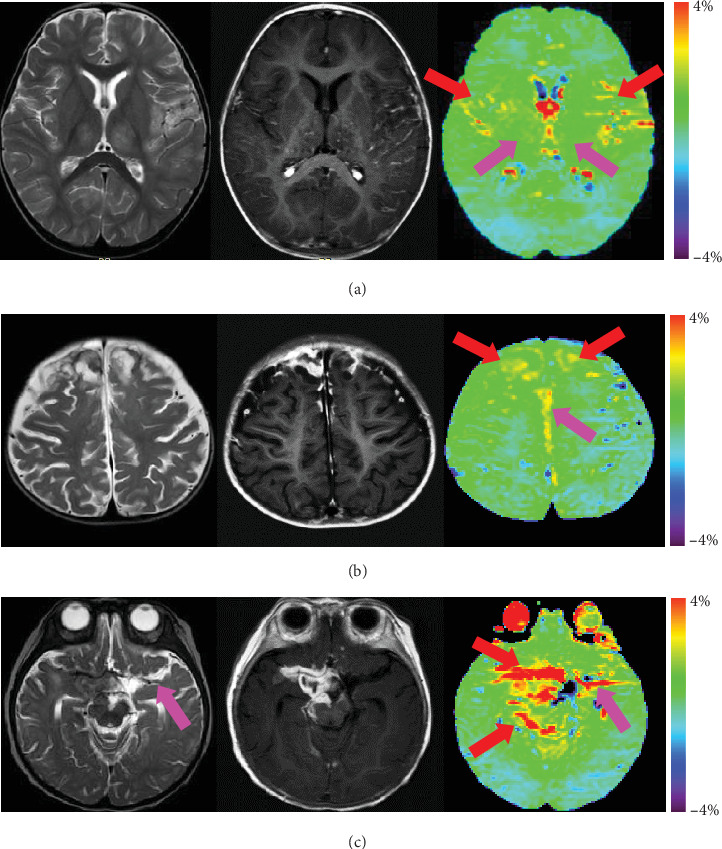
APTw and conventional MR images for three patients with meningitis. (a) T2w, Gd-T1w, and APTw images for a 17-month-old girl with viral meningoencephalitis. T2w image demonstrates symmetric increased signal intensity involving bilateral thalami and temporal lobes. The Gd-T1w image shows slight spotted enhancement in bilateral thalami and leptomeningeal enhancement in the bilateral temporal regions. The gadolinium-enhancing lesions (pink arrows) and leptomeninges (red arrows) show slight hyperintensity on the APTw image. (b) T2w, Gd-T1w, and APTw images for a 9-month-old girl with pyogenic meningitis. Gd-T1w image demonstrates leptomeningeal enhancement in the frontal regions and adjacent cerebral falx. Consistent with Gd-T1w, the APTw image shows hyperintensity in the frontal regions (red arrows) and adjacent cerebral falx (pink arrow). (c) T2w, Gd-T1w, and APTw images for a 7-month-old boy with tuberculous meningitis. Gd-T1w image shows enhancing subarachnoid space lesions in the right basilar cisterns, with extension into right sylvian vallecula, ambient cistern, and quadrigeminal cistern. The enhancing leptomeninges also show hyperintensity on the APTw image (red arrows). Note the APTw hyperintensity in the left sylvian vallecula and temporal lobe (pink arrow). T2w image verifies the presence of the left middle cerebral artery (pink arrow) in the same area.
